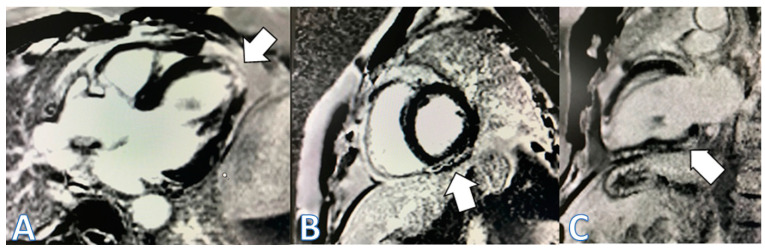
Cardiac MRI images. Left to right, (A) four-chamber view; (B) short-axis view; (C) two-chamber view. Late enhancement: intramyocardial and subepicardial patched foci in the anterolateral wall, basal-mid inferolateral and basal inferior segment, and intramyocardial foci at the mid-inferoseptal and apex segments (white arrows).
